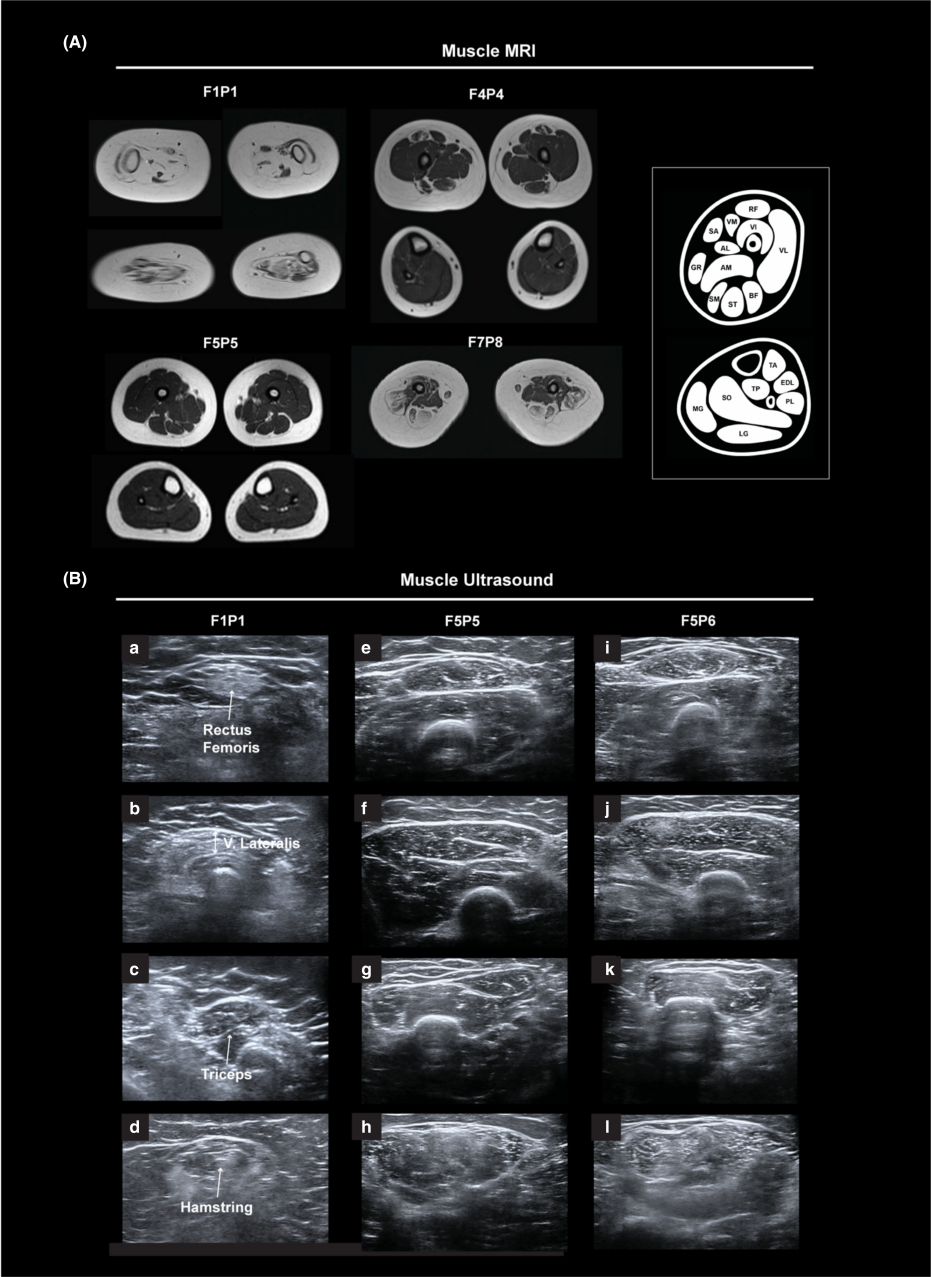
Muscle imaging in patients with biallelic *COL12A1* variants. (A) Muscle MRI, axial T1 at the thigh (top) and lower leg (bottom) for F1P1 (left, top) and F4P4 (middle, top), at age 15 months and 11 years, respectively. There was severe generalized muscle atrophy and fatty replacement in F1P1's proximal and distal lower extremity. Asymmetry and imaging artifact in the lower leg was due to contractures. F4P4's MRI demonstrated significant posterior thigh involvement with relative sparing of the semitendinosus. Preserved medial and anterior compartments of the thigh, with the exception of the rectus femoris with atrophy and fatty infiltrates noted around the central fascia. Muscle MRI in F5P5 (left, bottom) at age 6 years demonstrating normal appearance of muscles of the thigh and distal lower extremity. Muscle MRI of F7P8 (right, bottom) at age 7 years revealed relative sparing of the medial and anterior thigh compartments compared to the posterior thigh compartment, with selective atrophy of the rectus femoris, and to a lesser extent the vastus lateralis. Visualization of normal muscle imaging (far right). (B) Muscle ultrasound of F1P1 (age 15 months), F5P5 (age 6 years), and F5P6 (age 4 years) of the rectus femoris (RF) (a, e, and i), vastus lateralis (V. Lateralis) (b, f, and j), triceps (c, g, and k), and hamstring (d, h, and l). Imaging in P1 demonstrated diffuse muscle atrophy in the lower extremity (LE) more than upper extremity (UE) with dense granular increased echogenicity in the rectus femoris and vastus lateralis, and mixed pattern echogenicity in the triceps. Muscle ultrasound in F5P5 and F5P6 revealed mild–moderate granular/streaky echogenicity, most pronounced in the hamstrings and rectus femoris with no muscle atrophy.
